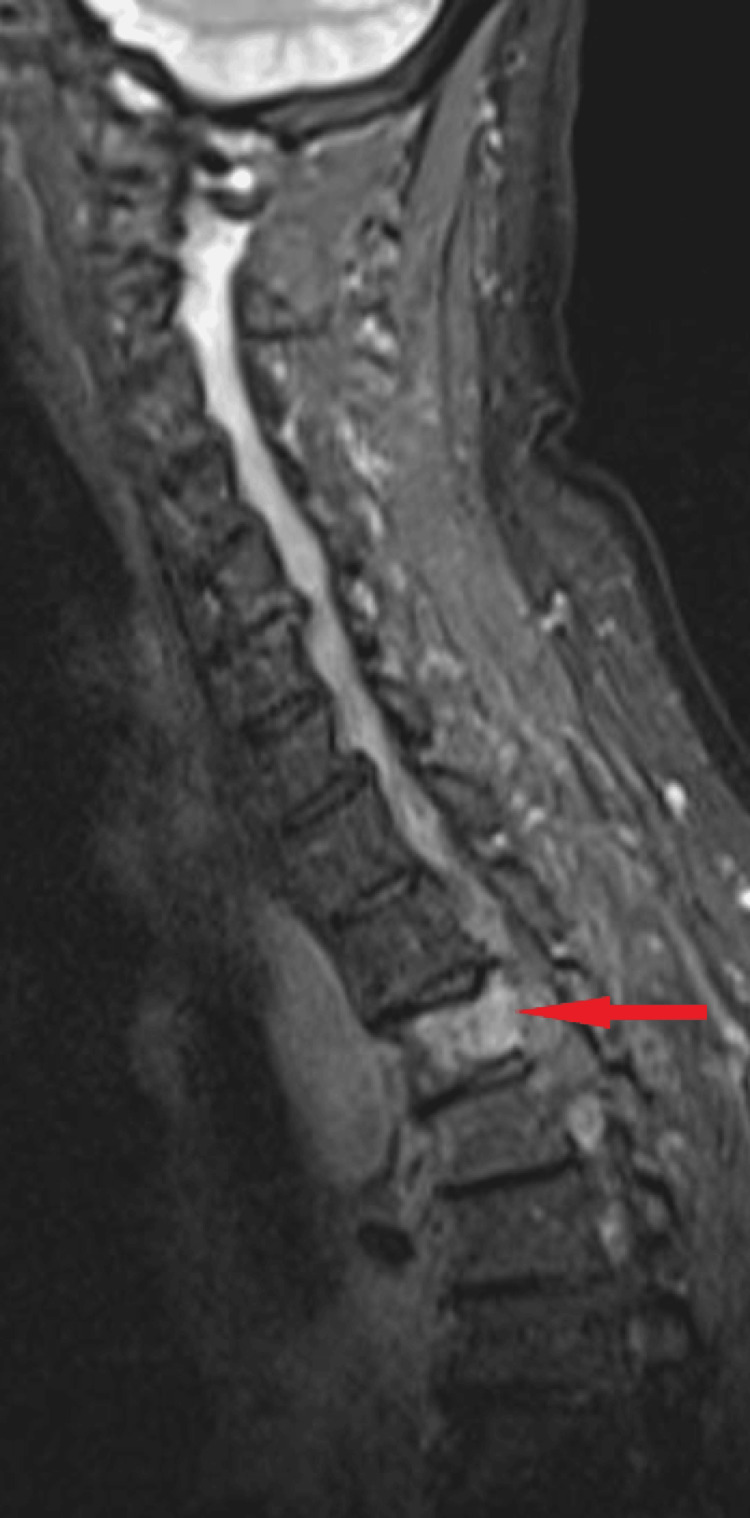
Magnetic resonance imaging of the spine. The red arrow points to an area of hyperintensity in the T3 vertebra. The report suggested that this was likely to be malignant infiltration with cord compression. There was a diffusely abnormal marrow signal throughout the cervical and thoracic spine on T1-weighted imaging.
